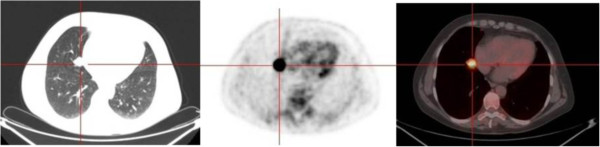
The integrated FDG-PET/CT reveals a small TC (20 mm) demonstrating high uptake (SUVmax 12.9). From left to right: CT, PET and fused image.
